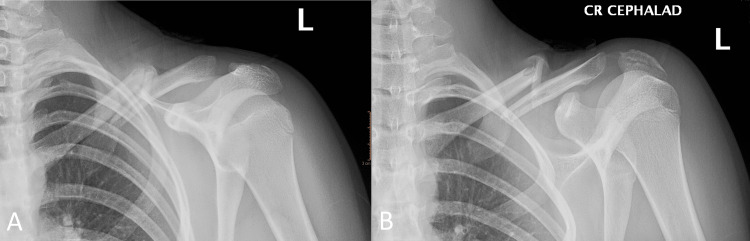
Left clavicle plain radiographs: anteroposterior and cephalad views demonstrate a comminuted and inferiorly displaced (lateral section) mid-shaft clavicular fracture with an approximate 3 shafts width of bayonet opposition and an approximate 1 ½ shaft width of inferior displacement (A and B)
